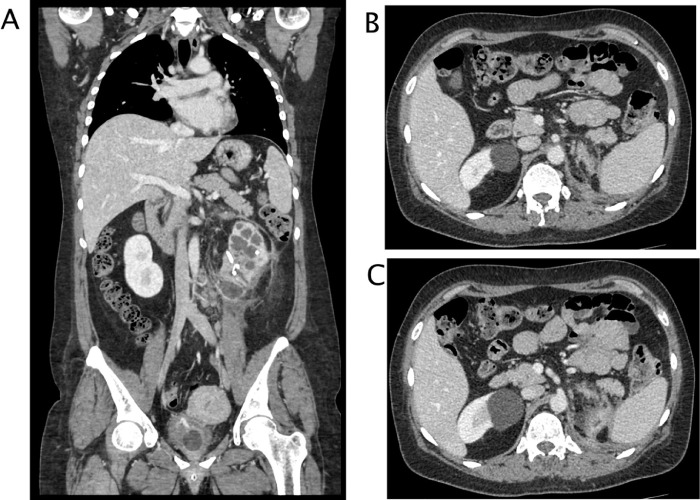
CT with intravenous contrast, parenchymal phase. Follow up CT after left nephrostomy and 15 days of antibiotic therapy. (A) multiplanar coronal plane reconstruction,.. The left calico-pelvic system is significantly decompressed, nephrostomy tube is appreciable in the pelvis. Perirenal fascia and fat are still thickened (B,C) axial images. On the upper pole of the left kidney the fluid component of the perinephric abscess is almost completely reabsorbed, a soft tissue with moderate enhancement is visible over the previously reported fistulous connection.
